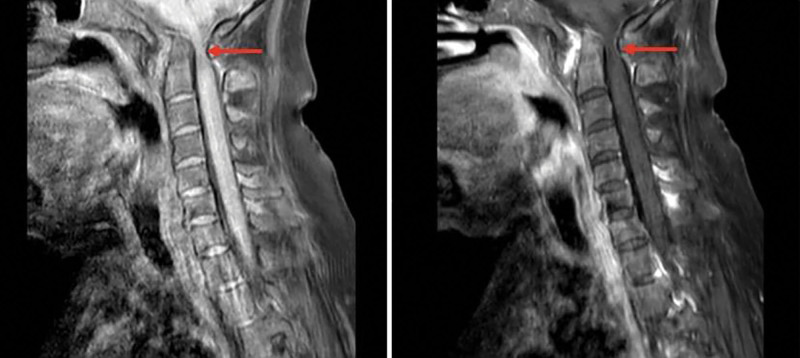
Cervical spine (enhanced) MRI: basilar invagination, plaque-like edema in the marrow; synovium thickening and swelling in the atlantodental space and some intervertebral facet joints, enhanced scan was obviously enhanced, adjacent bone mass erosi. MRI = magnetic resonance.
